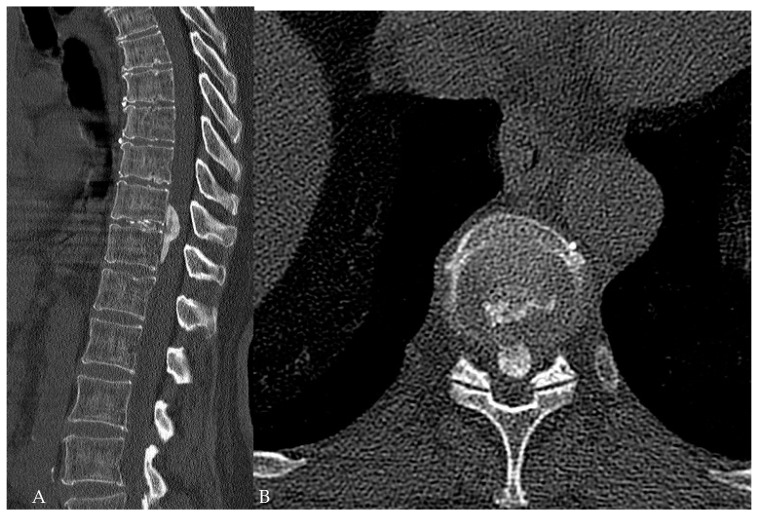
Patient No. 5, preoperative CT shows the herniated disc at the Th10–11 level with ossification of the posterior longitudinal ligament in the (A) sagittal and (B) axial view.
